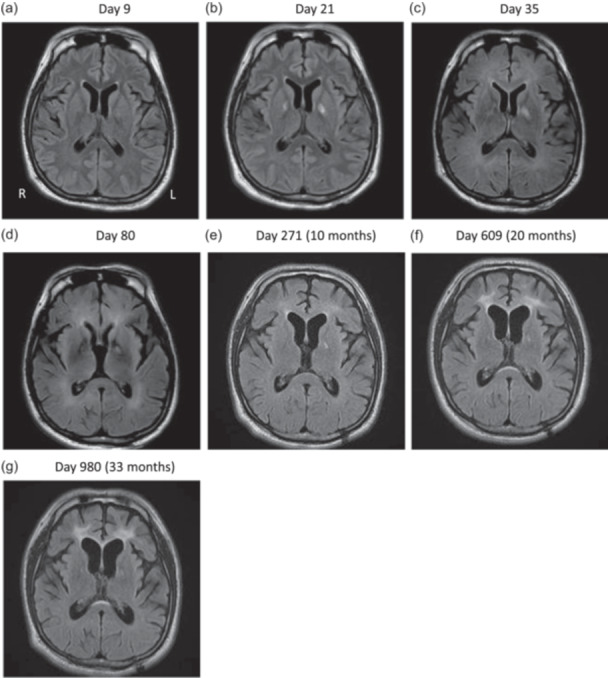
T2‐weighted fluid‐attenuated inversion recovery (T2 FLAIR) magnetic resonance (MR) images. Images (a) to (d) and (e) to (g) were taken with different MR scanners. Legions in bilateral globus pallidus were detectable at Day 9, most prominent at Day 21 and less in later images. Anterior horn was widening during the whole course supported by Evans index (0.276 at Day9, 0.294 at 10 months, 0.319 at 20 months, and 0.332 at 33 months). High intensities in periventricular and centrum semiovale white matter appeared on Day 35, and thicker periventricular white matter lesions in frontal lobes were found at 20 and 33 months.
